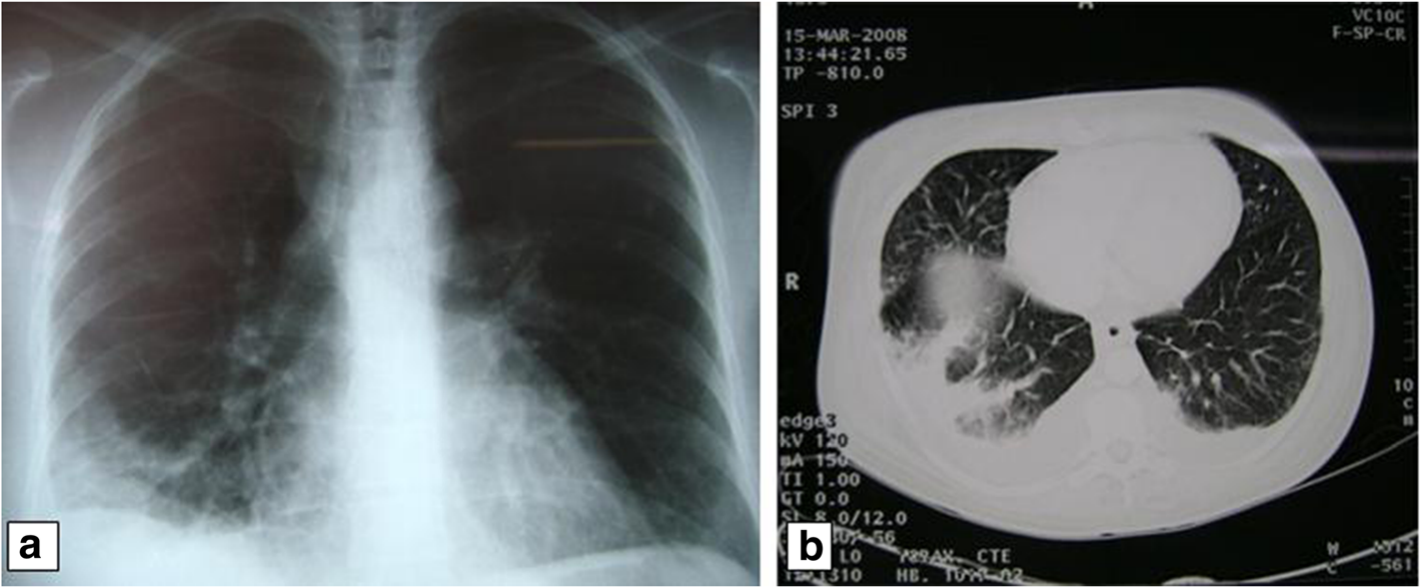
Unusual Paraclinical findings of AOSD in lung. A chest radiograph shows a patchy right lobe consolidation in the lower lung zone (a). CT image shows extensive areas of airspace consolidation due to alveolar hemorrhage in right lung. The bilateral pleural effusions are also notable (b).
